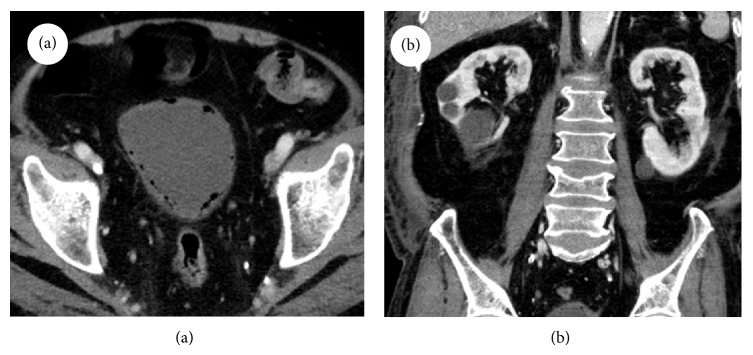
(a) Computed tomography of the abdomen showed multiple diffuse gas collection in the bladder wall suggestive of emphysematous cystitis. (b) Both kidneys and psoas muscle were unremarkable except multiple cortical cysts.
